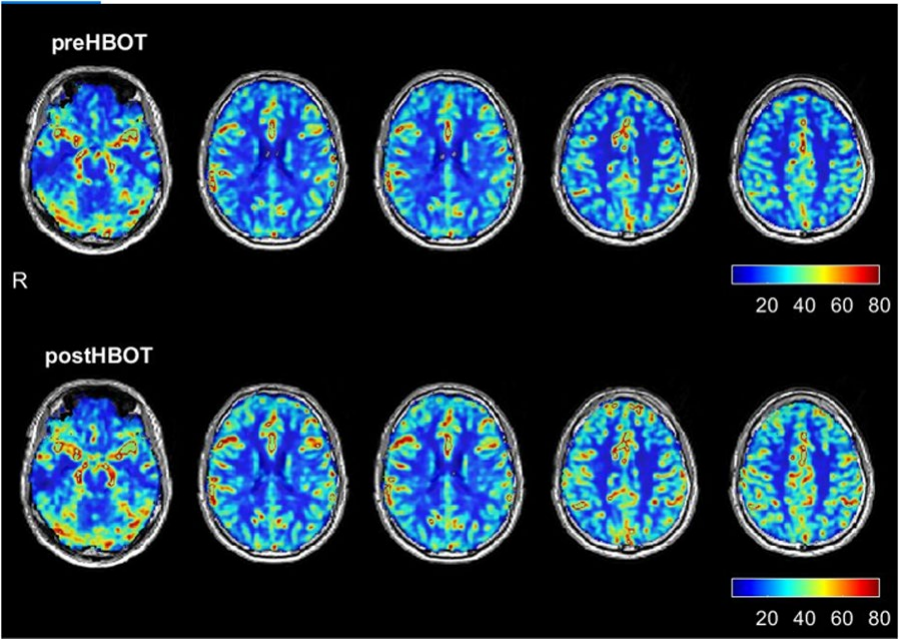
Brain perfusion magnetic resonance imaging before and after hyperbaric oxygen therapy. The upper row represents brain perfusion 3 months after the acute infection, before hyperbaric oxygen therapy. The lower row represents the perfusion magnetic resonance imaging done after completing the hyperbaric oxygen therapy protocol
